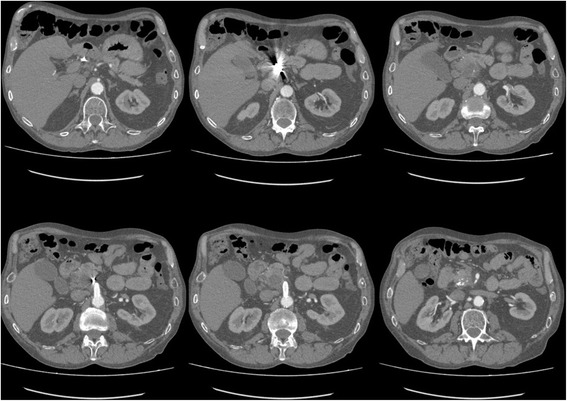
Control computed tomography angiography after 6 months shows complete thrombosis and resorption of the pseudoaneurysm. Covered stent in the superior mesenteric artery and coils in the common hepatic artery
